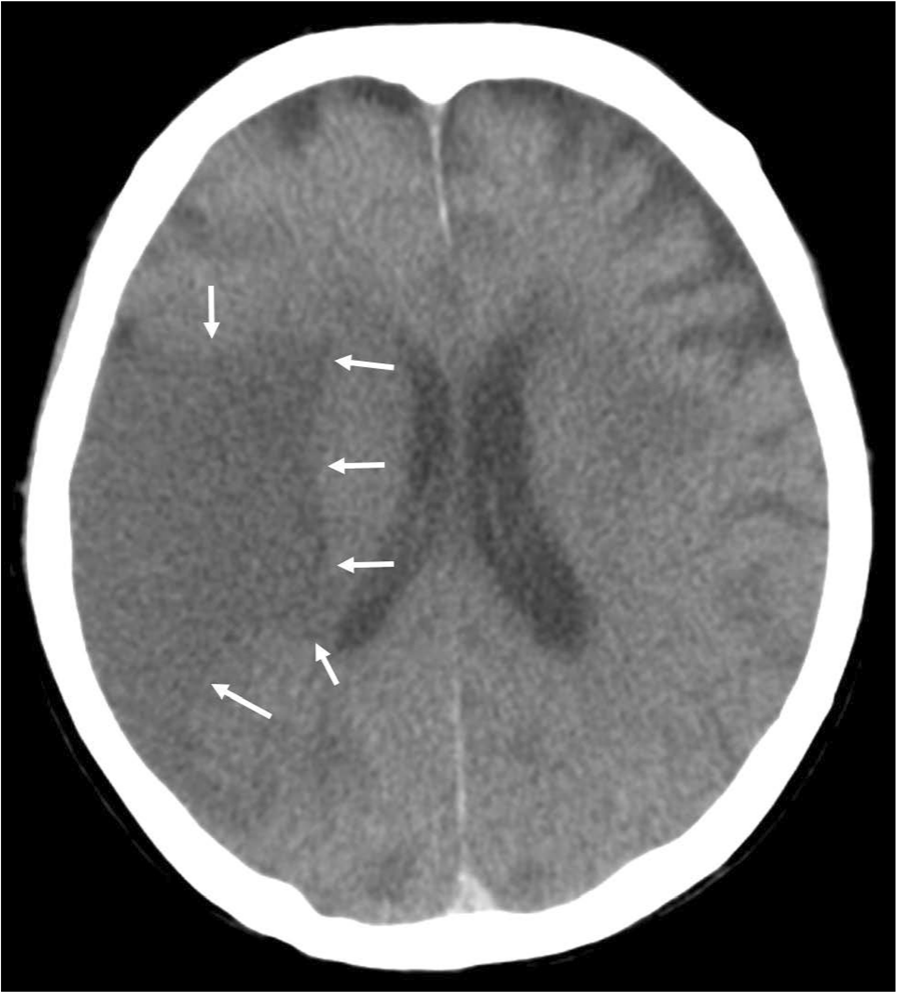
Brain postmortem CT image 14 h after death (Case 1). An LDA was found in the middle cerebral artery area (*arrows*)
